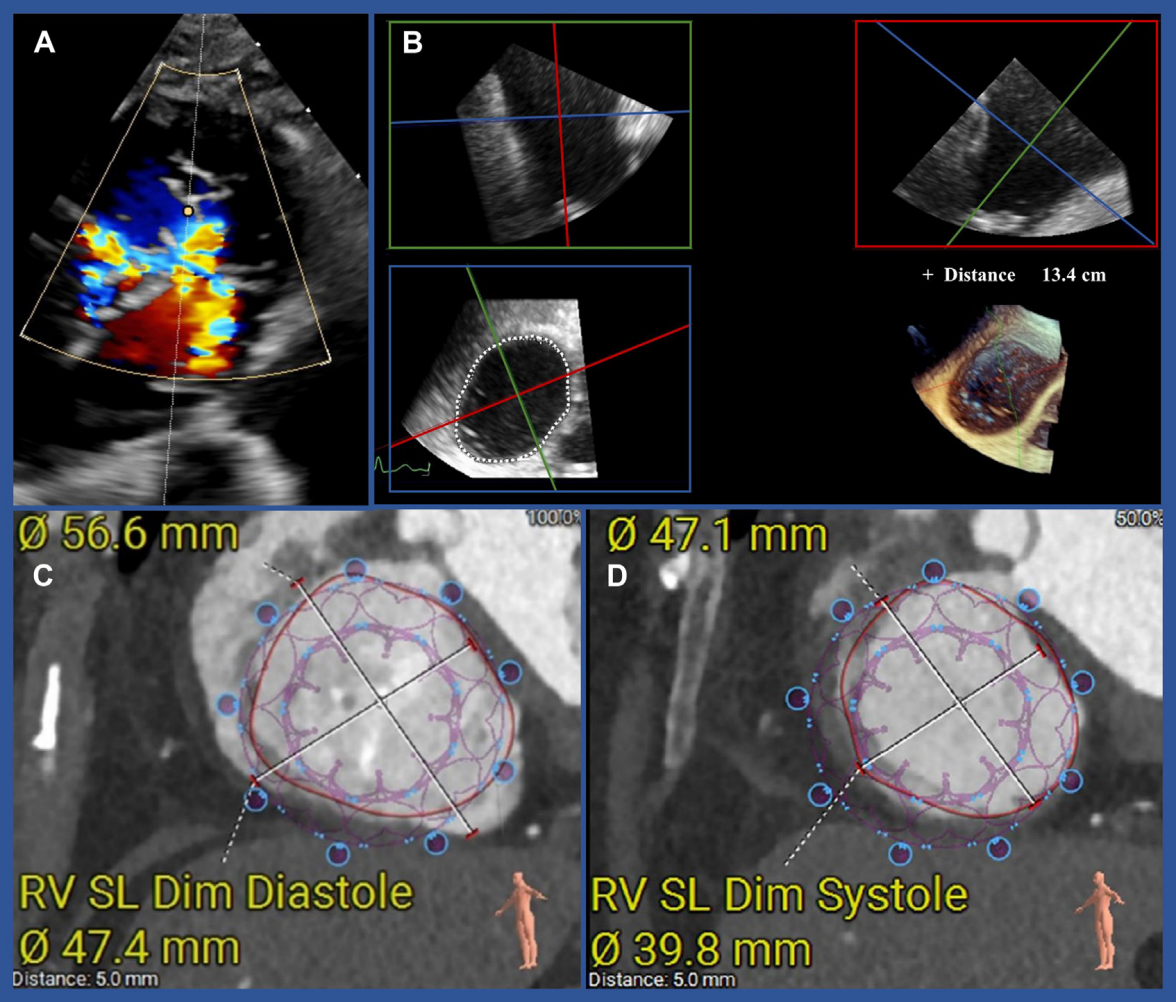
Screening for Transcatheter Tricuspid Valve Replacement (EVOQUE) (A) Torrential tricuspid regurgitation according to echocardiography. (B) Sizing the tricuspid valve using echocardiography and multiplane reconstruction. (C and D) Sizing the tricuspid valve using computed tomography. RV SL Dim = right ventricular septal lateral diameter.
